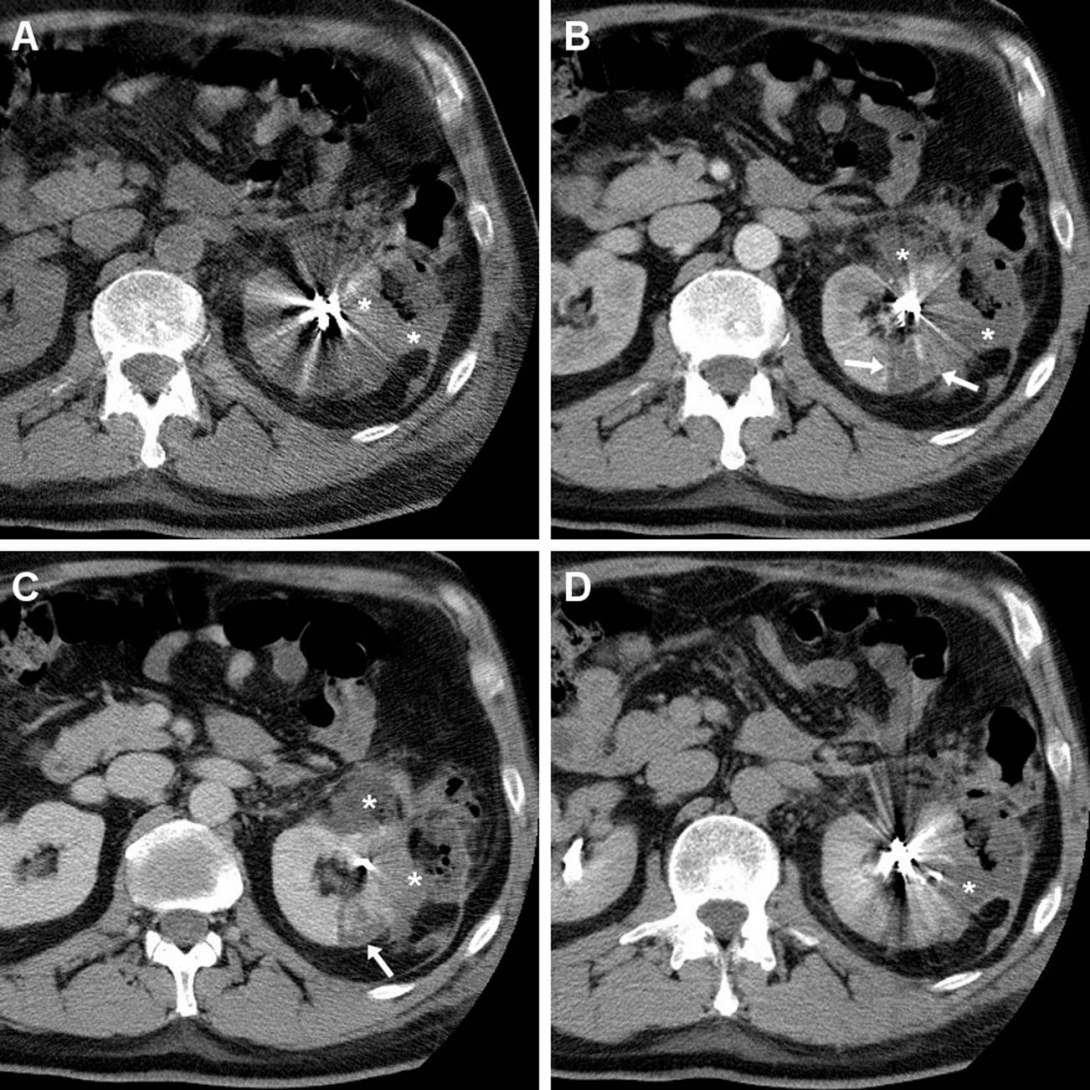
Post-embolisation status in a 53-year-old man with acute haemorrhage after L-PN. Selective embolisation of arterial branches with Embozene particles and metallic coils achieved bleeding control and prompt clinical improvement. Five days later, unenhanced (a), corticomedullary (b) and nephrographic (c) phase multidetector CT acquisitions showed strongly hyperdense coils at the renal sinus causing beam-hardening artefacts, some residual peri-renal blood (*) without persistence of active bleeding, and a wedge-shaped non-enhancing parenchymal area (*arrows*) consistent with focal infarction. Excretory (d) phase images excluded urine leak from the collecting system
